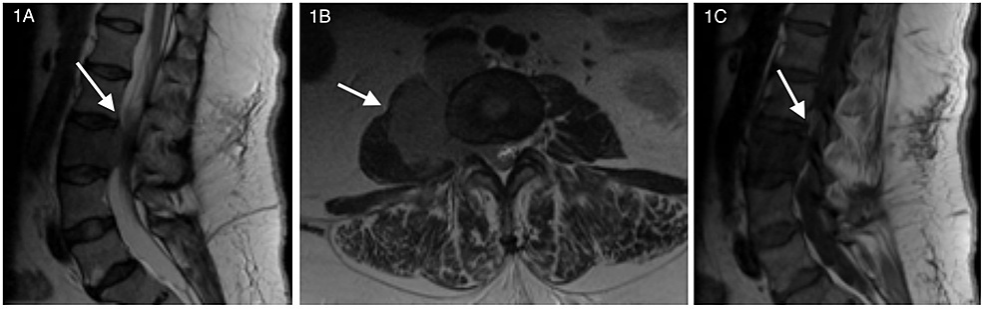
Noncontrast MRI of the lumbar spine. The image on the left (1A) is a sagittal T2 MRI of the lumbar spine, the middle image (1B) is an axial T2 MRI of the lumbar spine, and the image on the right (1C) is a sagittal T1 MRI of the lumbar spine. Interpretation: L3 vertebral body mass with prevertebral (7.5 cm x 4.2 cm x 6.1 cm) and epidural (2.1 cm x 1.2 cm x 4.5 cm) components. There is an evident retroperitoneal mass invading L3 vertebral body and extending into the epidural space with the epidural component of the mass resulting in severe compression of the thecal sac/cauda equina.
